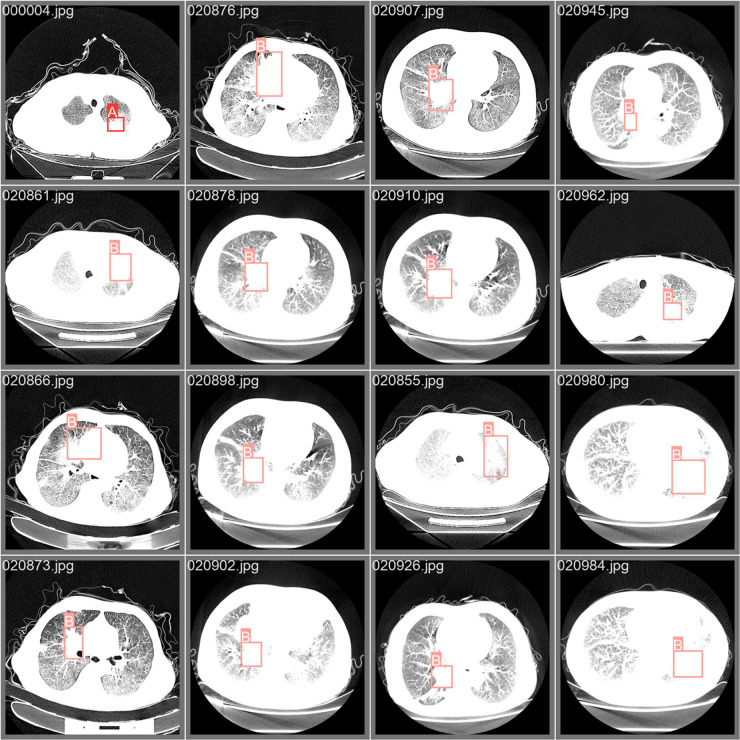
YOLO-ED detection effect on LUNG-PET-CT-DX.
